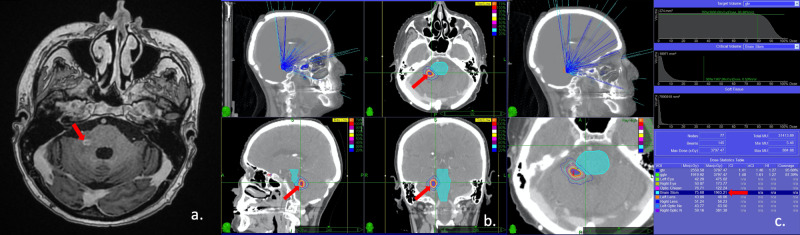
MRI of the lesion and treatment planning images a. Axial contrast-enhanced magnetic resonance imaging (MRI) scan of the new lesion in the right middle cerebellar peduncle. b. Axial, sagittal, and coronal view of the treatment planning CT scan of the lesion in the right middle cerebellar peduncle. The orange line is the prescribed isodose (79%). c. Dose-volume histogram for the lesion in the right middle cerebellar peduncle (red arrow shows the maximum dose of the brain stem).
